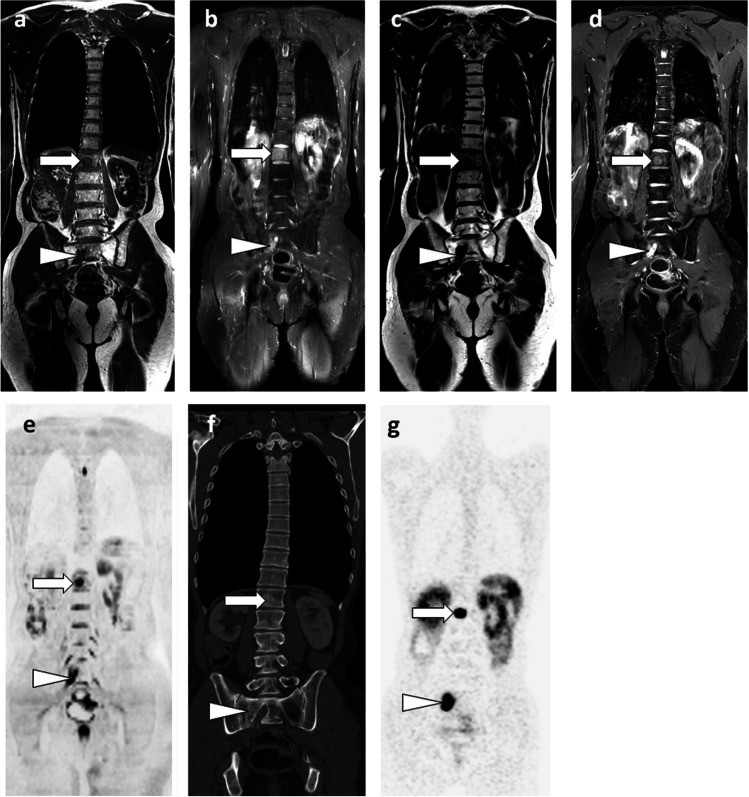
46-year-old man with newly diagnosed neuroendocrine cancer: comparison of T1 and STIR sequences with a single T2 Dixon sequence, DWI and PET/CT. a, b Coronal T1 and STIR WB-MRI sections show two metastases within the L1 vertebral body (arrow) and the right sacral wing (arrowhead). c, d Fat-only (FO) (c) and water-only (WO) (d) images of the corresponding FSE T2 Dixon sequence, show exactly the same lesions (in a single sequence). e Coronal high *b*-value (*b* = 1000 s/mm^2^, inverted grayscale) DWI sequence shows the same lesions. f, g Gallium-68 dotatate PET/CT (octreo-PET) show the same lesions, slightly sclerotic on CT (f) and showing intense tracer uptake (g)
